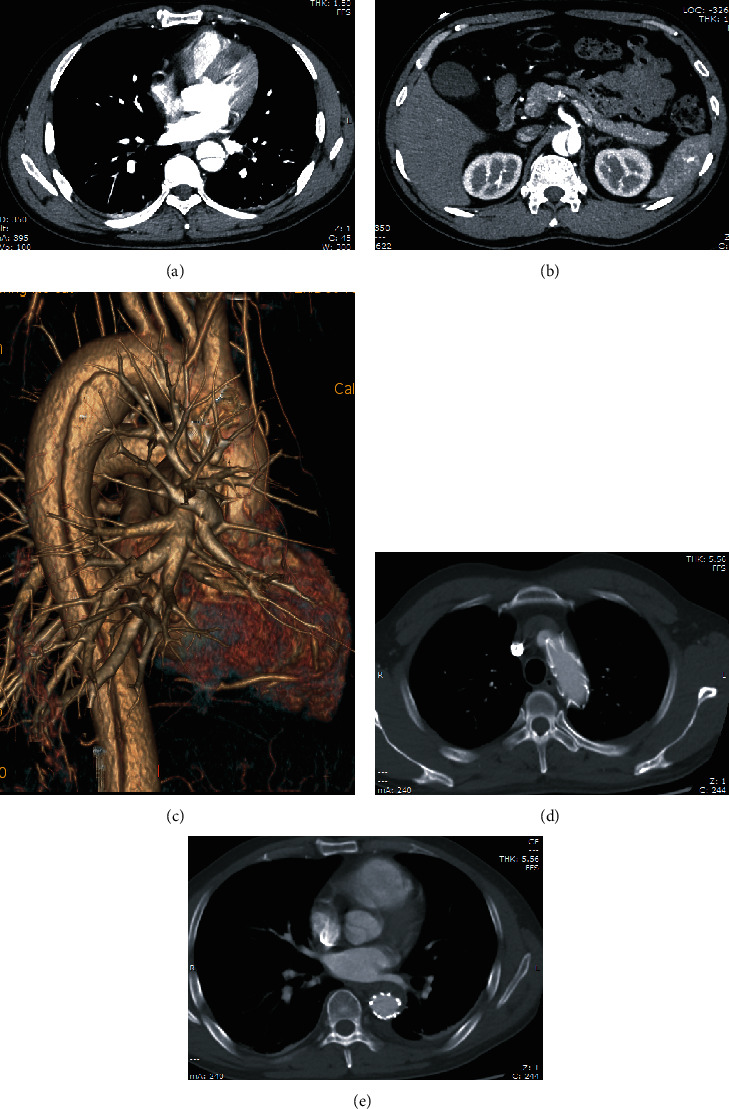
(a) CT angiography scan revealed double-lumen aortic arch structure. (b) CT angiography scan showed the double-lumen structure of the abdominal aorta. (c) CT three-dimensional imaging suggested aortic dissection. (d and e) After aortic stenting, no internal leakage was visible.
